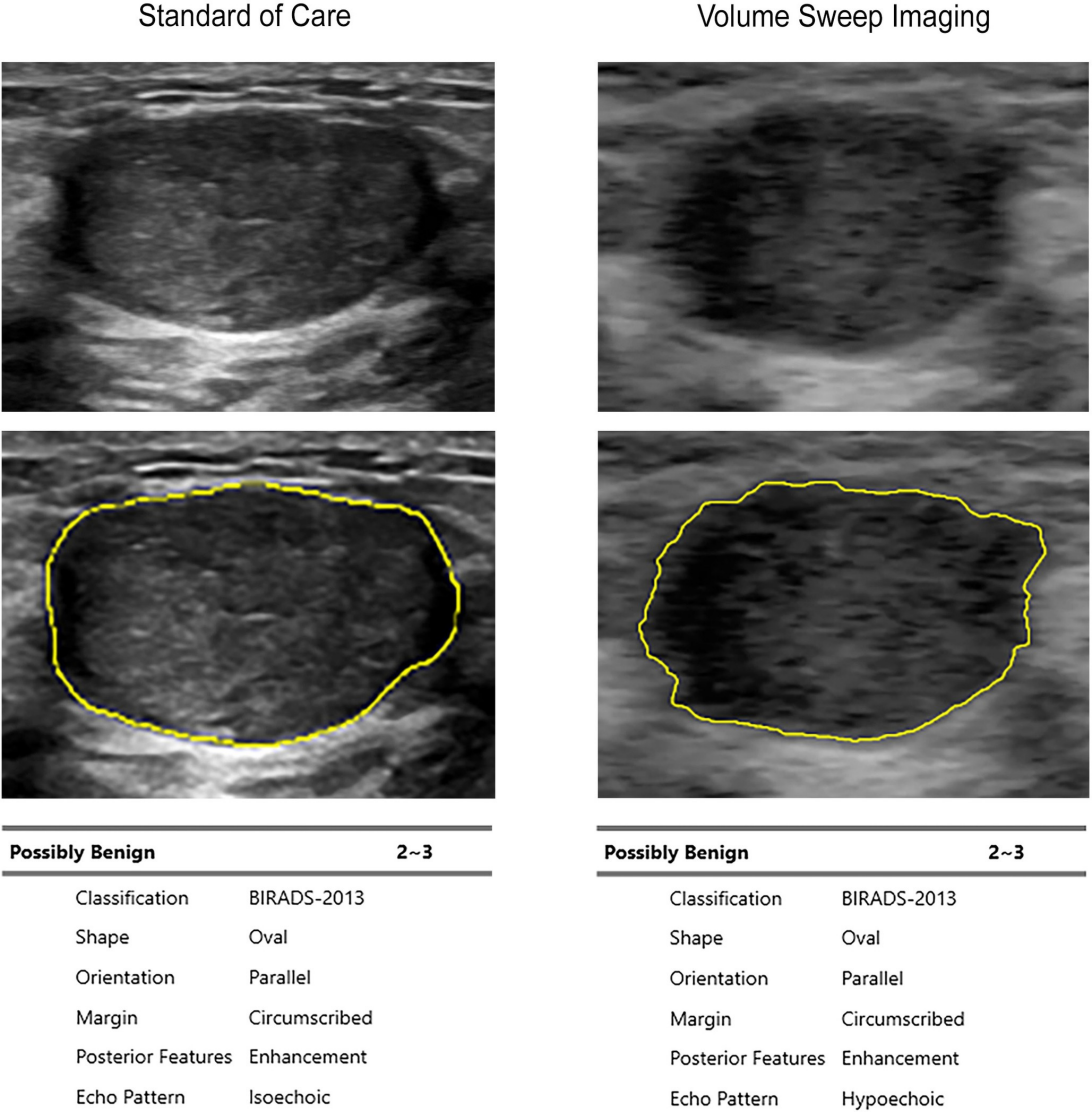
S-Detect Interpretation of a Fibroadenoma on Standard of Care and VSI. B-mode ultrasound images of a fibroadenoma are shown with their respective mass segmentations and S-Detect produced reports. The standard of care image was obtained by a certified expert breast sonographer using a General Electric Logiq E10 ultrasound machine. The VSI image was obtained by a medical student without prior medical experience after less than 2 hours of training on a portable Butterfly iQ ultrasound probe. S-Detect results between standard of care and VSI are identical apart from standard of care echogenicity reported as isoechoic (a trivial difference). The S-Detect results for VSI were identical to expert interpretation of standard of care and VSI.
